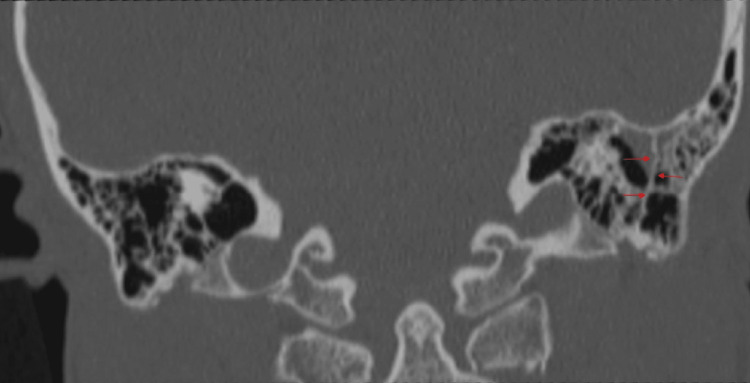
HRCT temporal bone: coronal section with right ear showing the presence of Korner's septum (red arrows)
